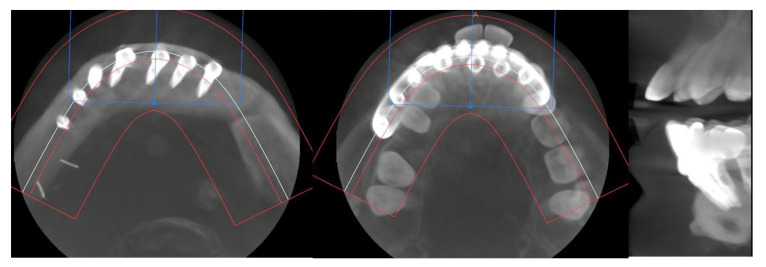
CBCT performed in the follow-up to evaluate bone resorption before and after implant loading.
